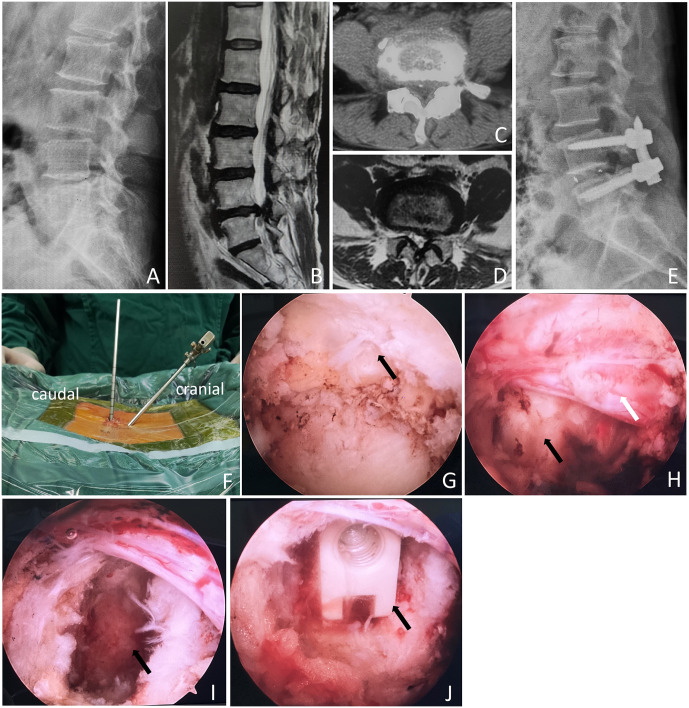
A patient with lumbar disc herniation (LDH) accompanied by spinal stenosis who underwent UBE-LIF. A showed the preoperative lumbar lateral X-ray. B showed LDH with spinal stenosis on the L4/5 in preoperative lumbar sagittal MRI. C and D showed the presence of LDH with spinal stenosis on the L4/5 axis, respectively. E showed the internal fixation after UBE-LIF was in good position after surgery. F showed the observation tube (cranial) and working tube (caudal) of UBE-LIF. G showed the endoscopic removal of the ligamentum flavum (black arrow). H showed endoscopic exposure of the nerve root (white arrow) and herniated disc (black arrow). I showed the endoscopic removal of intervertebral discs (black arrow). J showed the cage (black arrow) was implanted endoscopically.
